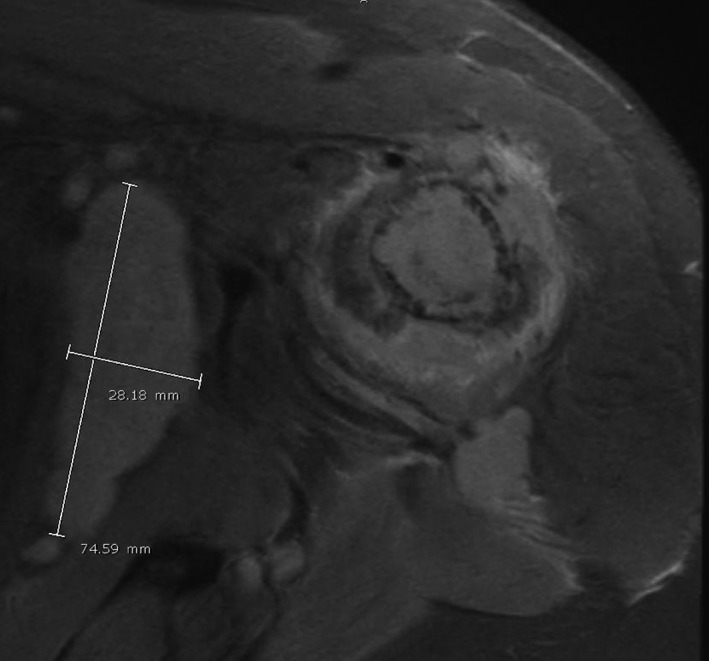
MRI of the left shoulder (coronal view) shows a large tumor replacing the marrow of the proximal humerus with an aggressive‐appearing periosteal reaction and cortical destruction. There is also a nodal mass in the left axilla anterior to the subscapularis muscle measuring approximately 6.4 × 7.5 × 2.8 cm
